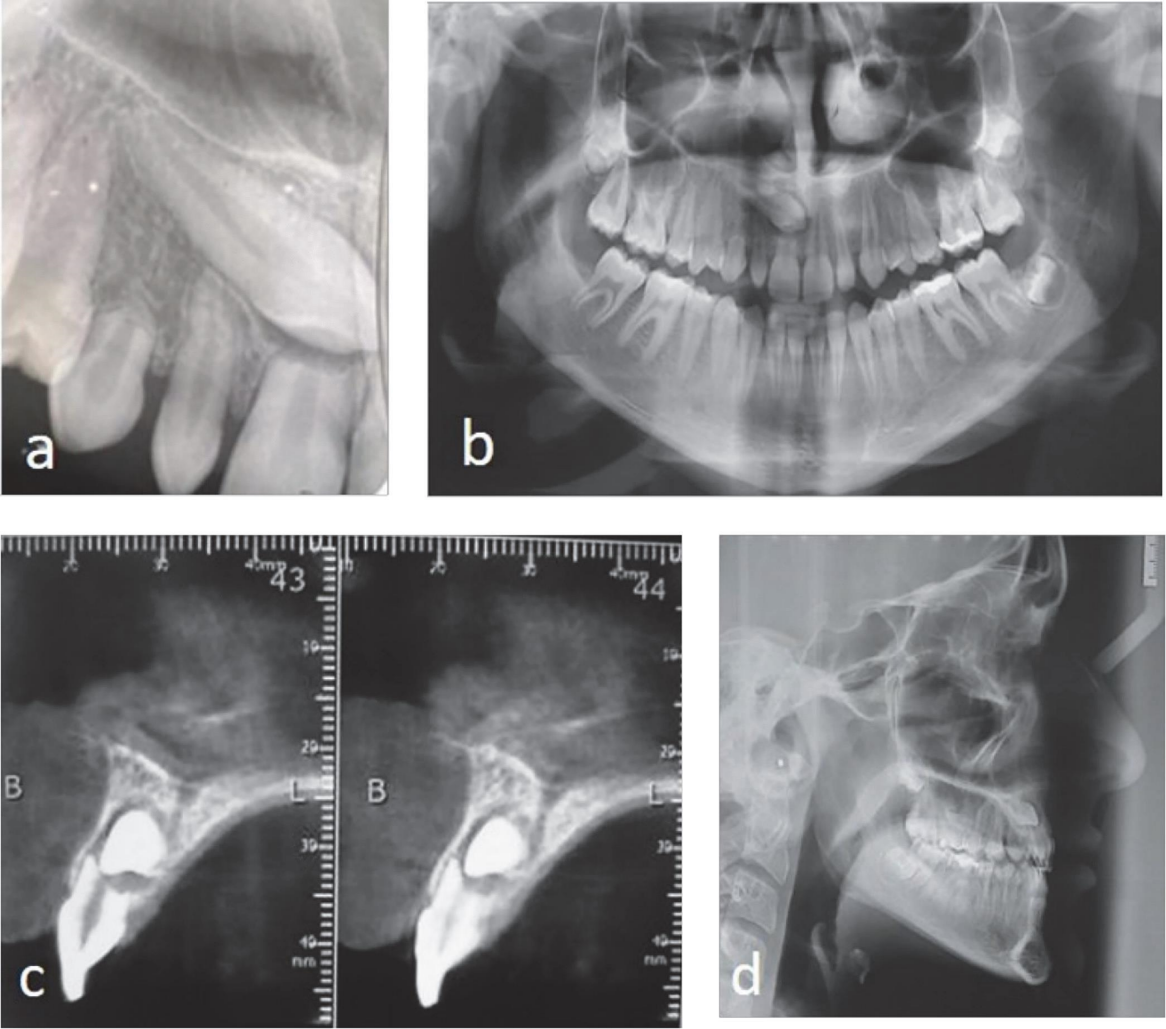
Preoperative radiographic examination: (a) periapical radiograph; (b) panoramic radiograph; (c) cone‐beam computed tomographic scan; and (d) cephalometric radiograph.
